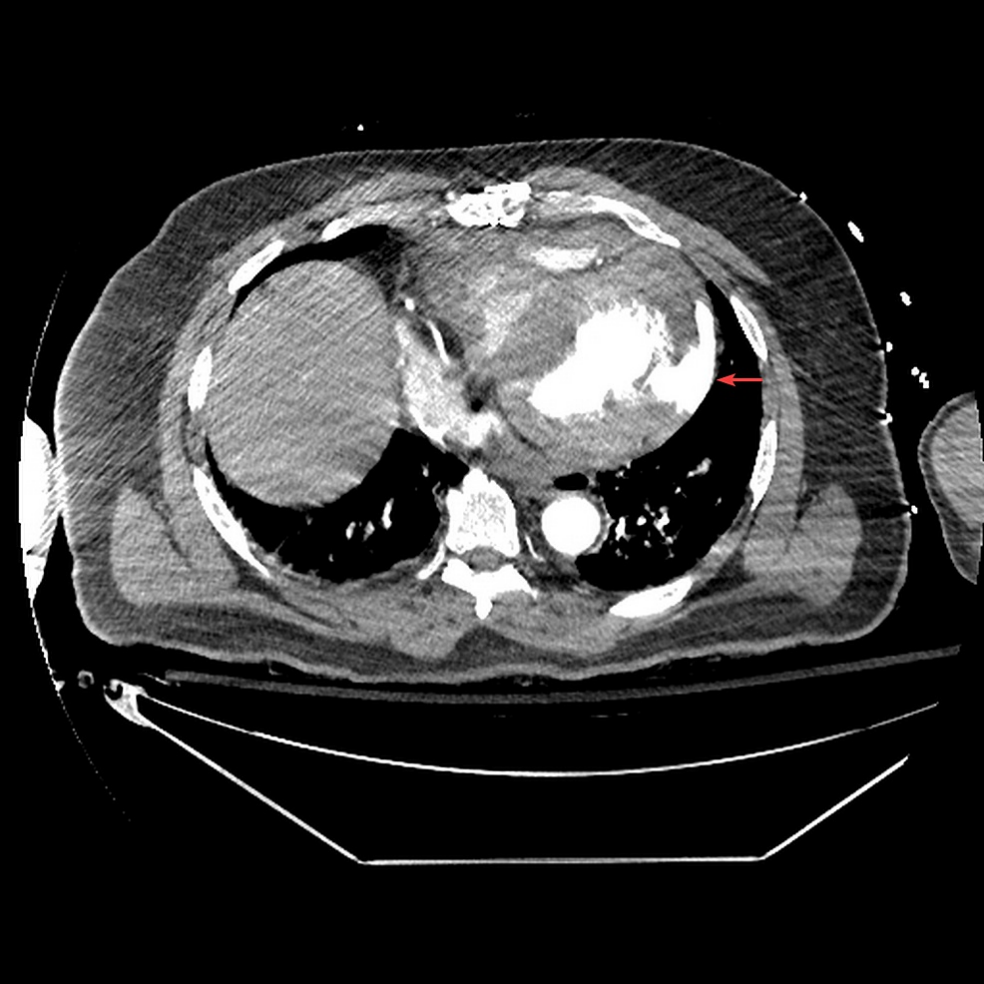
Transverse view of computed tomography with angiography of the chest showing a ventricular free wall rupture with extravasation of contrast from the left ventricle to the pericardial space (red arrow).
